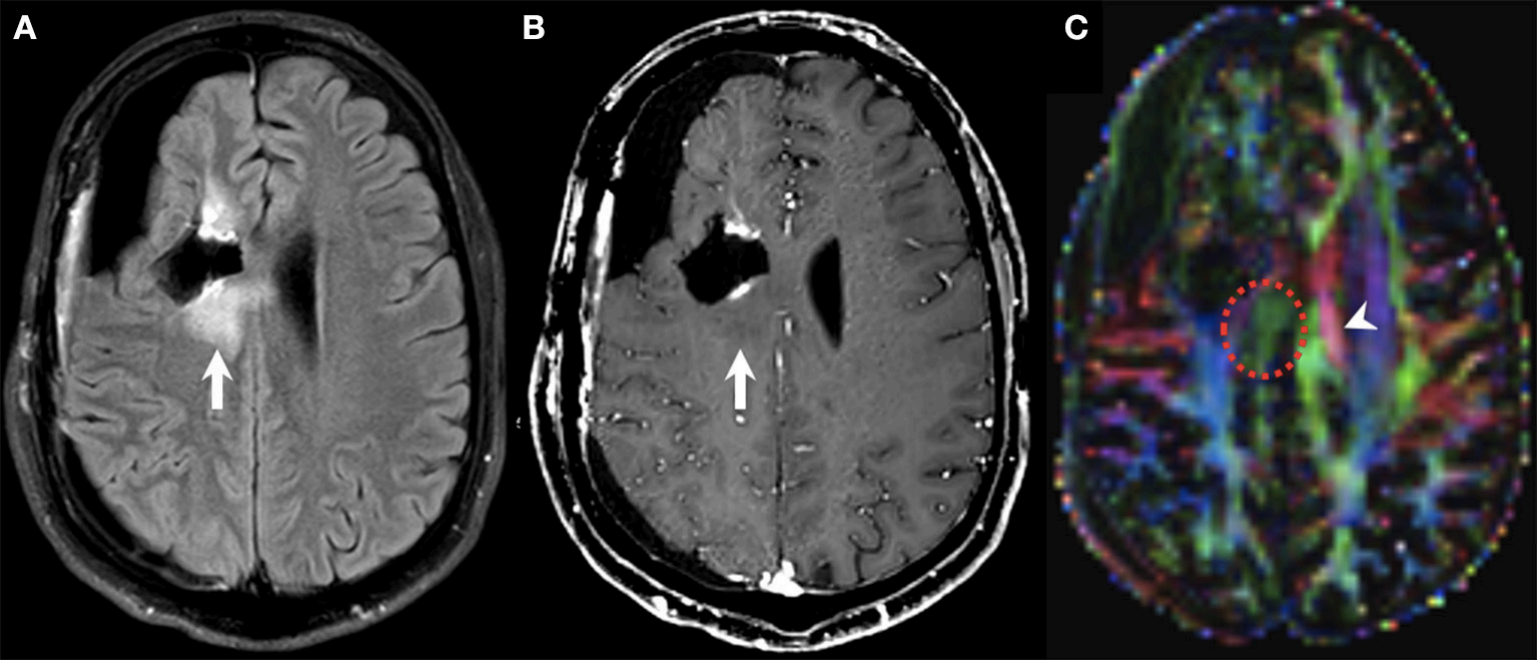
Diffusion tensor imaging (DTI) in pseudoresponse. Follow-up brain MRI of a 30-years-old male person with a diagnosis of GBM (NOS, MGMT status unknown) after standard-of-care treatment, currently on bevacizumab therapy. FLAIR (A), contrast-enhanced T1w (B), and DTI (C) axial images. A new area of non-enhancing FLAIR hyperintensity is noted along the posterior aspect of the right frontal surgical cavity (arrows on A,B) that was considered as infiltrative tumor based on other functional MRI sequences (not shown). DTI unveils the disruption of the corpus callosum fibers subjacent to the lesion (dotted circle on C) compared to the normal contralateral side (arrowhead on C) as a marker of tumor infiltration.
